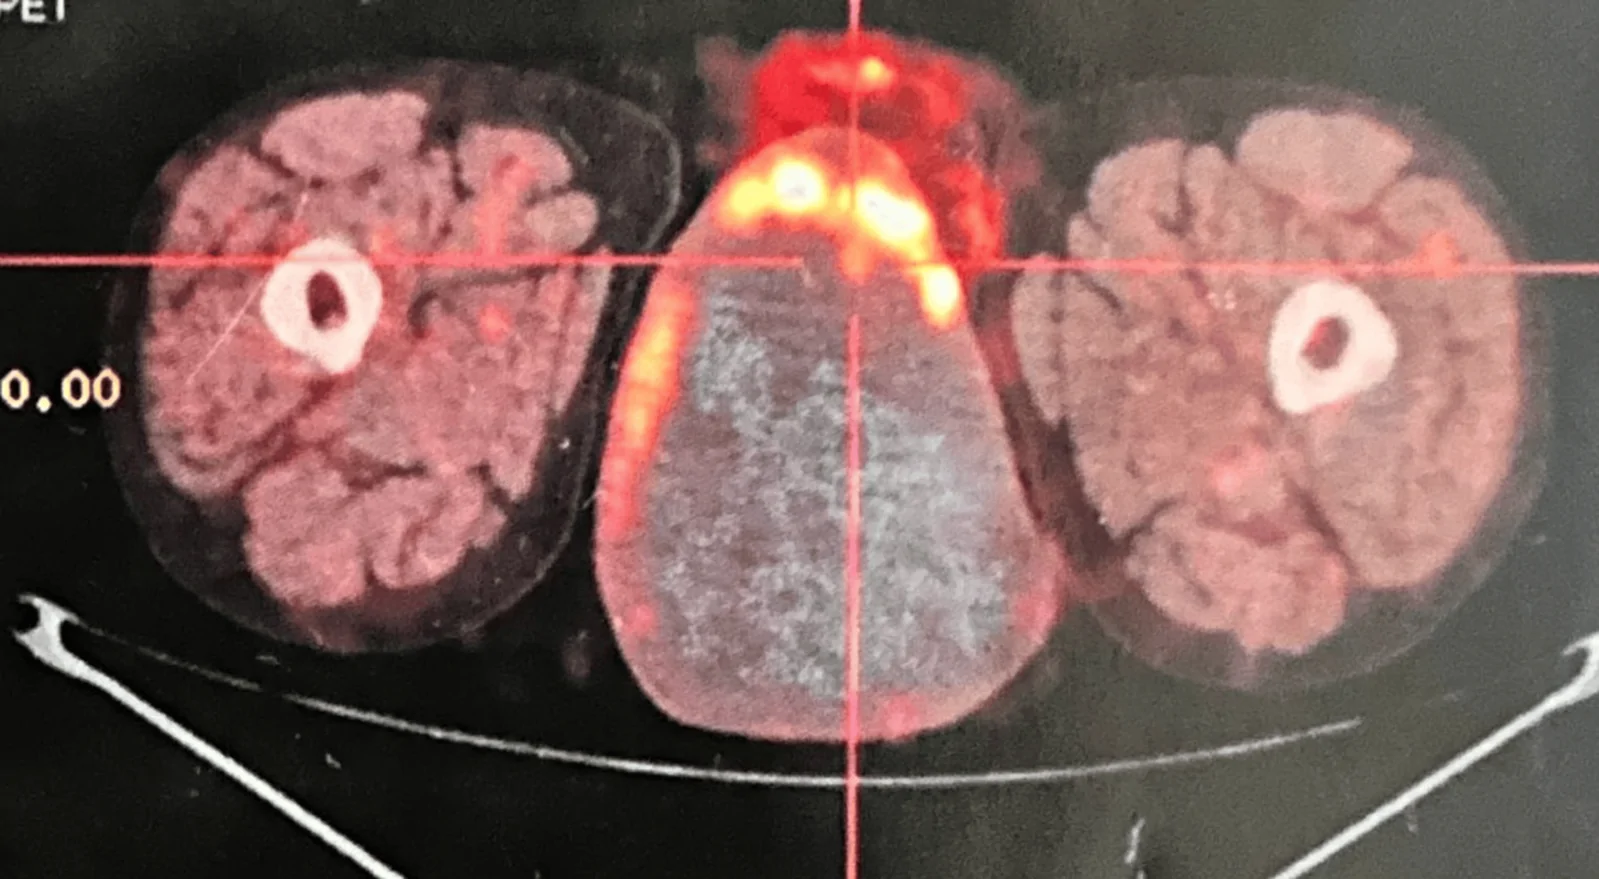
Axial prostate-specific membrane antigen (PSMA) PET-CT revealing a solitary, intensely hypermetabolic left intratesticular focus (SUVmax 18).
